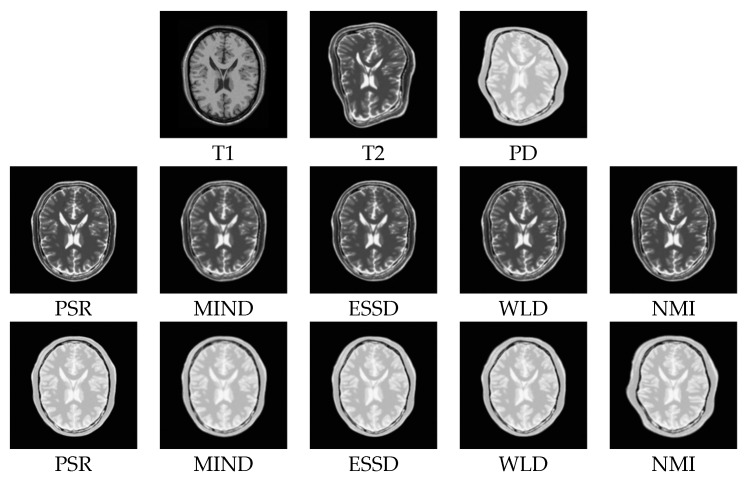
Registration results for the PSR, MIND, ESSD, WLD, and NMI methods performed on the BrainWeb dataset (T1–T2, T1–PD). The first row corresponds to the T1-weighted, T2-weighted, and PD-weighted MR images, respectively. The second row corresponds to the registered results of T1–T2 images for PSR, MIND, ESSD, WLD, and NMI, respectively. The third row corresponds to the registered results of T1-PD images for PSR, MIND, ESSD, WLD, and NMI, respectively.
